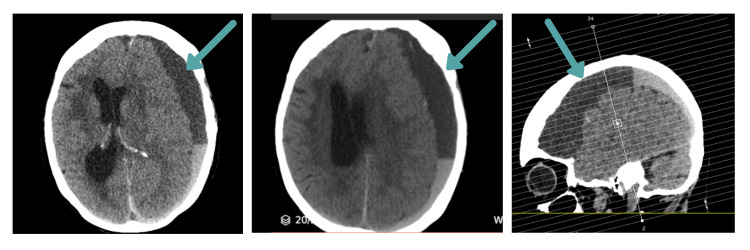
CT head showing rebleeding after COVID-19 infection CT demonstrating worsening acute-on-chronic left subdural haematoma measuring 16 mm with new layering indicating acute rebleeding. Midline shift increased to 12 mm (from 9 mm).
